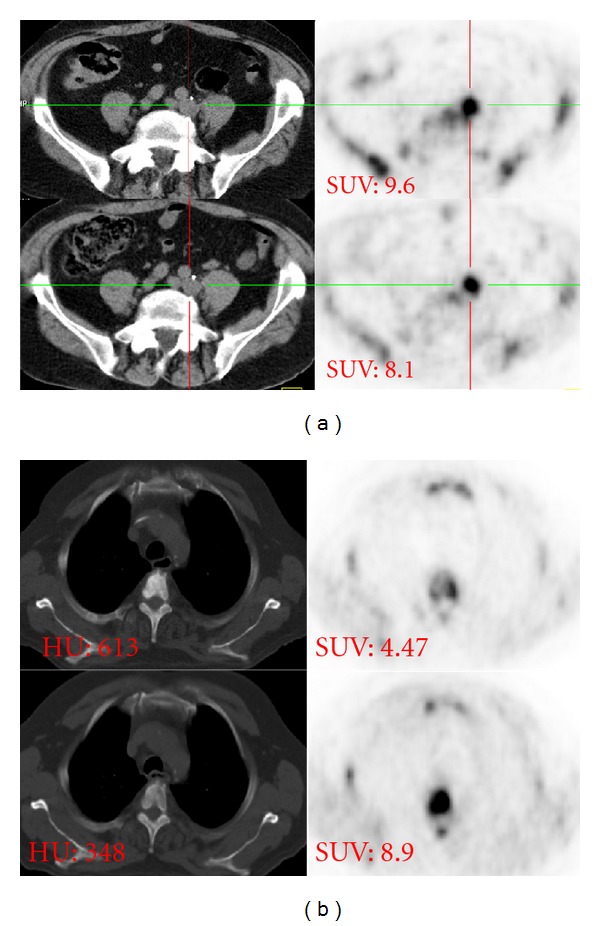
73-year old patient with prostate cancer metastatic to regional lymph nodes (a) and thoracic spine (b). Lower row: baseline Upper row: followup after 6 months under AHT. Bone metastasis (b) shows a dramatic SUV (max) decreasing (8.9 versus 4.47); however, the associated CT shows an increasing of sclerosis degree (HU 348 versus 613) suggesting progressive disease in spite of SUV decline. The lymph node metastasis (a) shows a SUV(max) rising (8.1 versus 9.6), confirming thus the progress.
